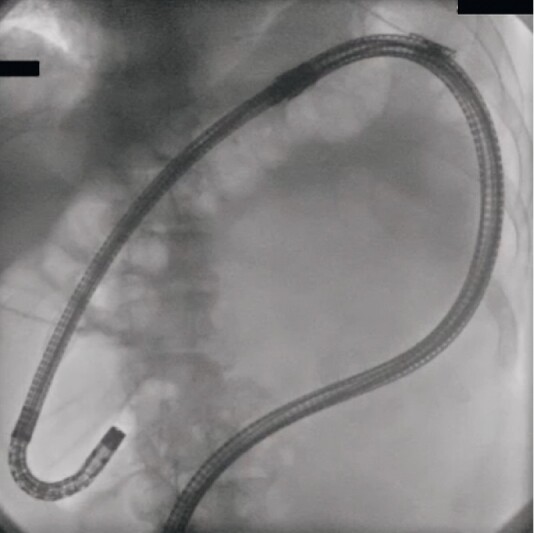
Abdominal radiograph during endoscopic submucosal dissection showing no migration of gas into the downstream colon, and a straight position of the scope.
